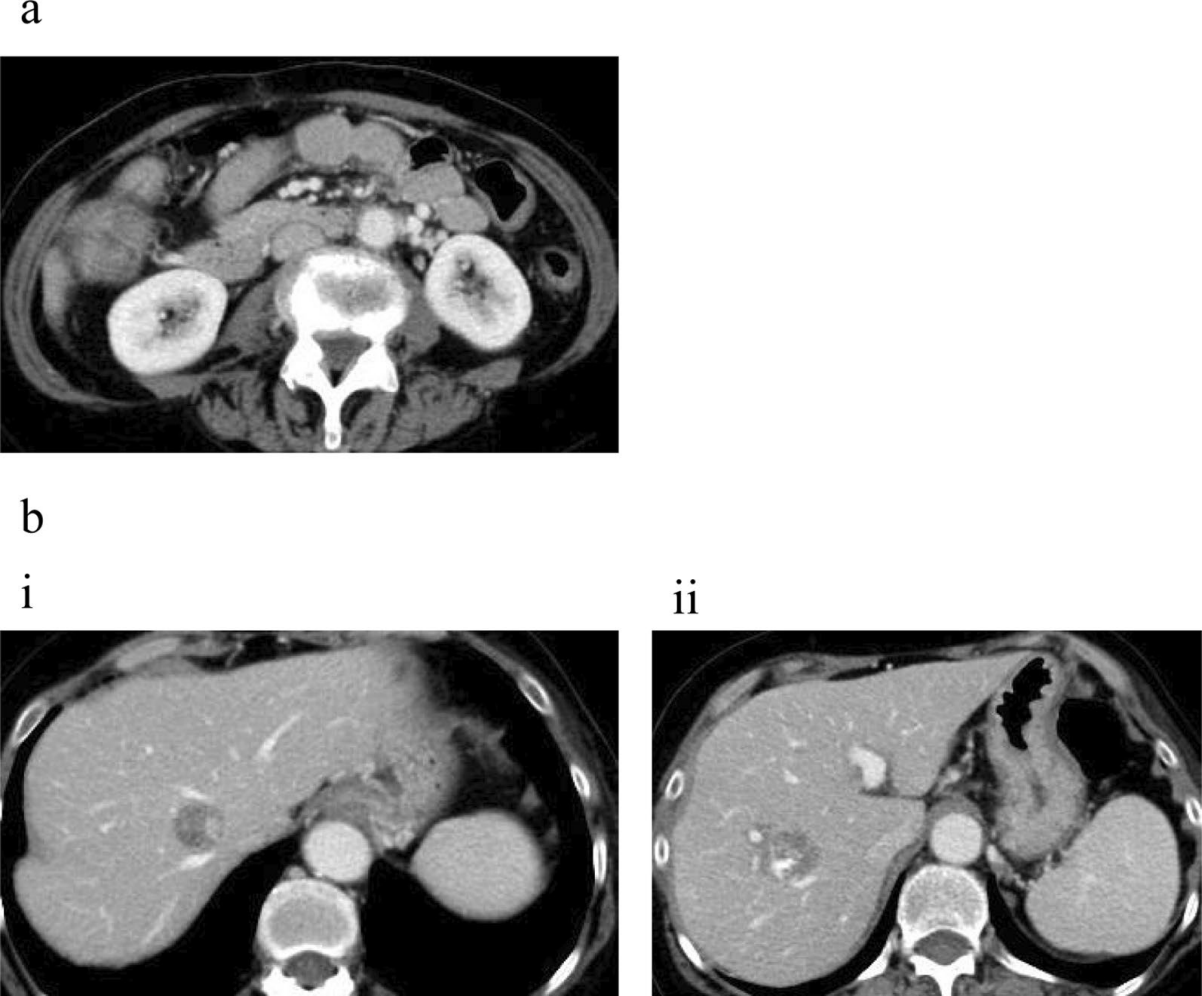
Computed tomography images after preoperative chemotherapy.
